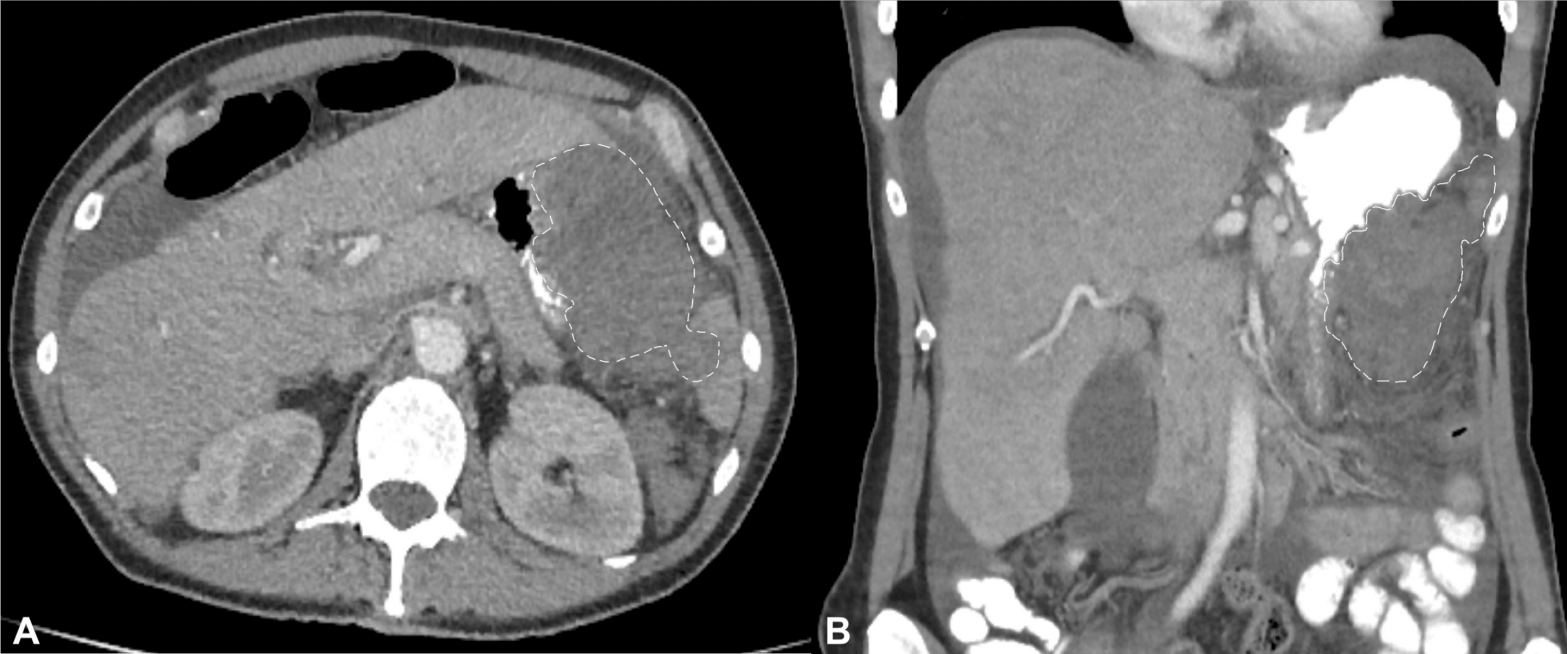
Contrast-enhanced CT of the abdomen (A) Axial and (B) coronal images demonstrate a well-circumscribed, heterogeneously enhancing soft-tissue mass (outlined) arising from the greater curvature of the stomach. The lesion measures approximately 11 cm, abuts the splenic hilum, and displaces the transverse colon inferiorly.
